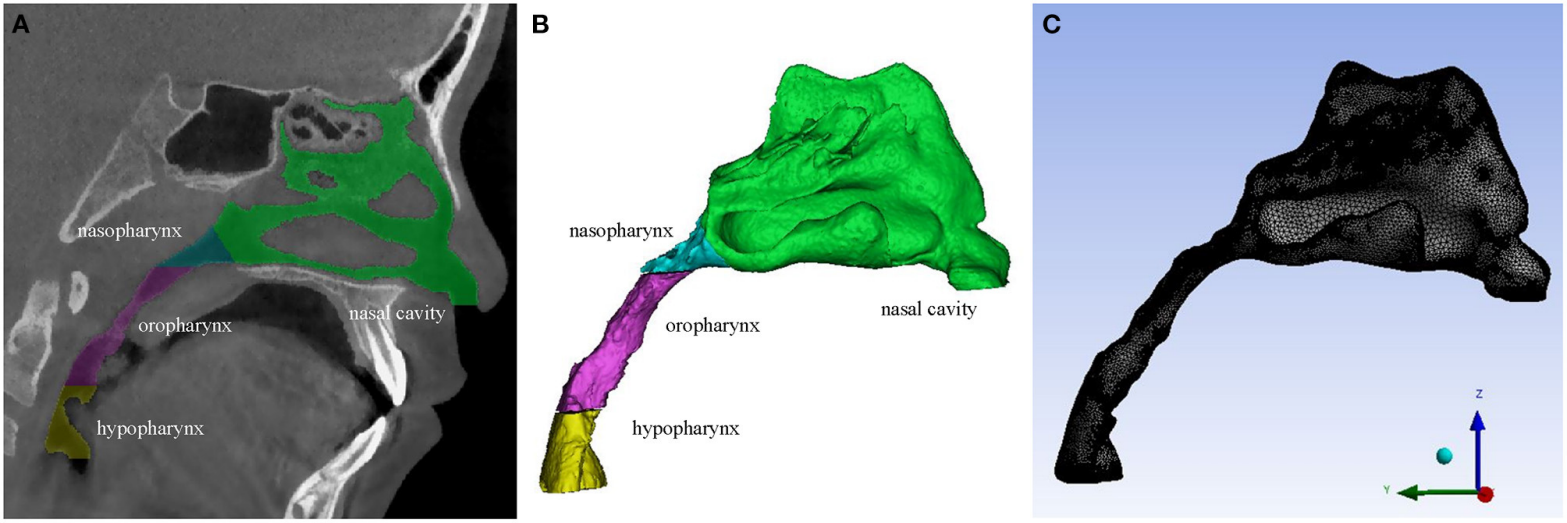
(A) A CBCT slice of the upper airway. (B) 3D model of the upper airway. (C) Mesh generation of the upper airway 3D geometry.
